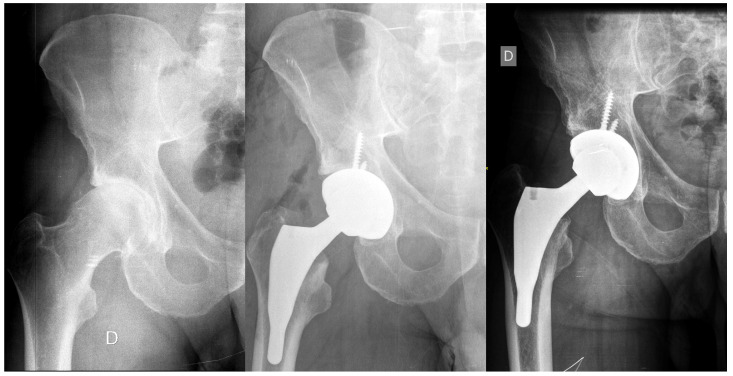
AP X-rays displaying the (I) preoperative condition, (II) immediate postoperative status, and (III) 5-year follow-up of a patient treated with an Evolution hip stem.
